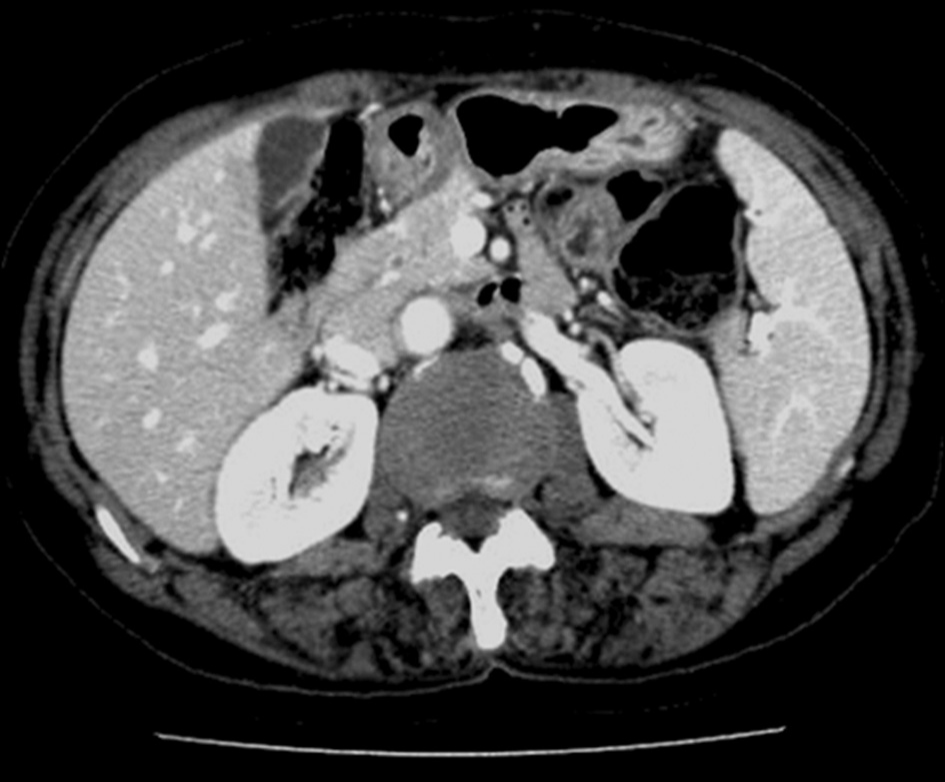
Computed tomography, after six month course of anti-tubercular therapy, demonstrates low density nodules and ascites disappeared completely.
